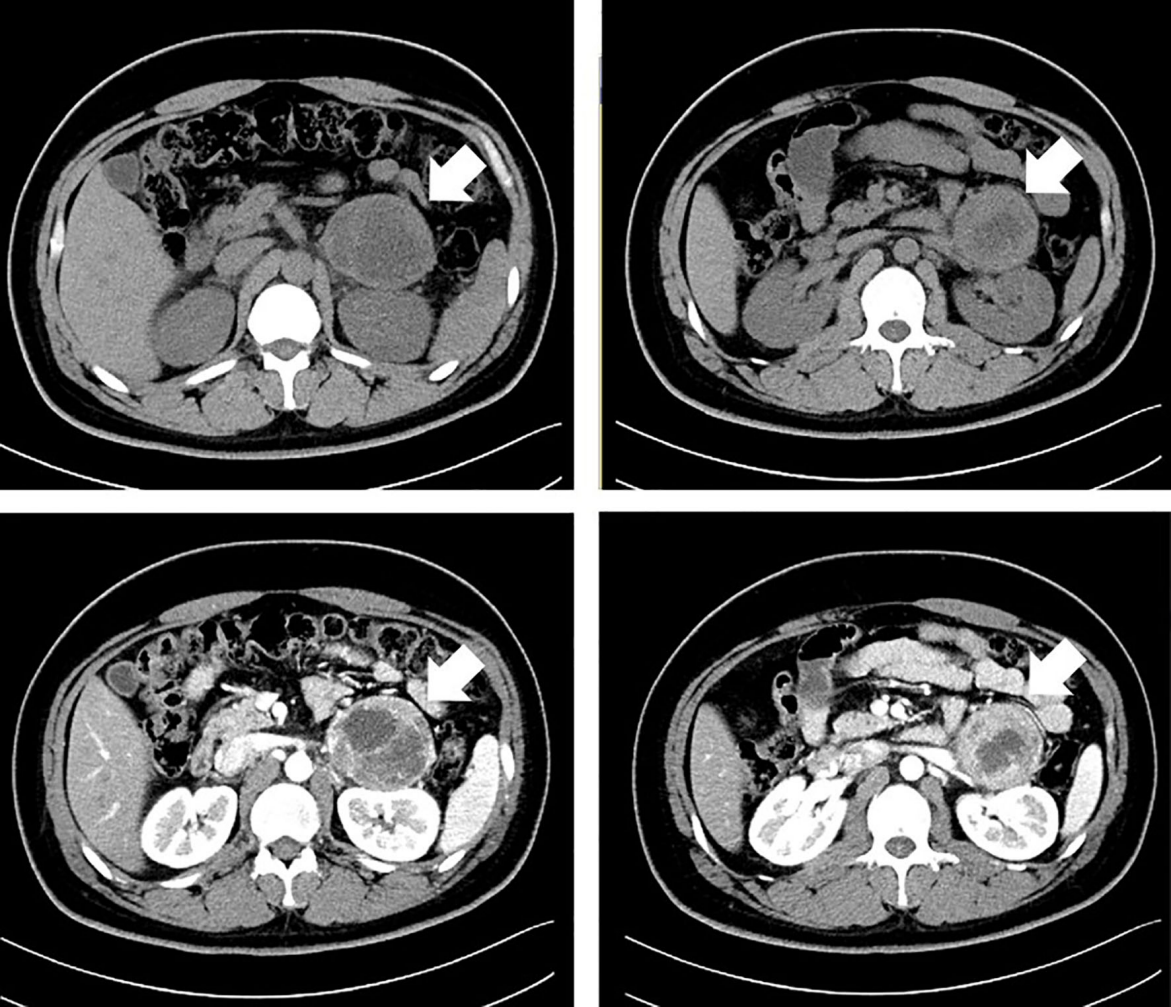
A computed tomographic (CT) scan of the abdomen with intravenous contrast in the patient with a left adrenal mass.
